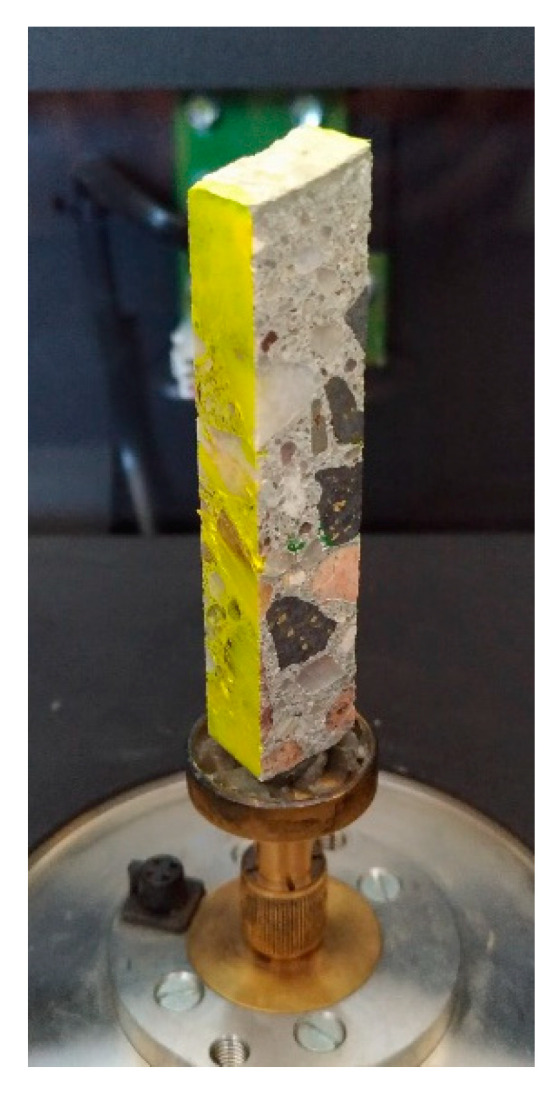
The sample attached to the holder and mounted in the chamber of X-ray scanner.
